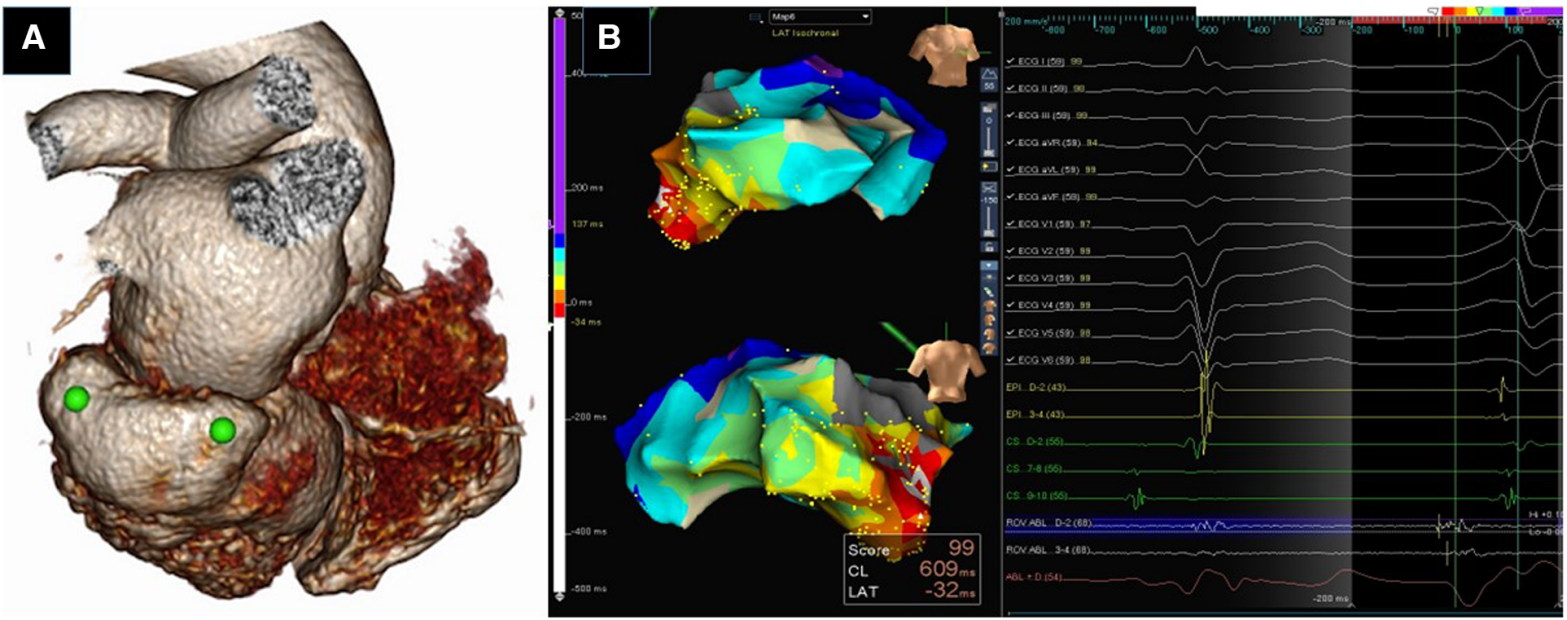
Utility of Pre-procedural imaging. Three dimensional volume rendered CT image of heart showing submitral posterobasal aneurysm (green dots) (A). 3D Electroanatomic Images in RAO and PA showing earliest activation in the aneurysm (white arrow) (B). RFA at this site eliminated the PVCs.
